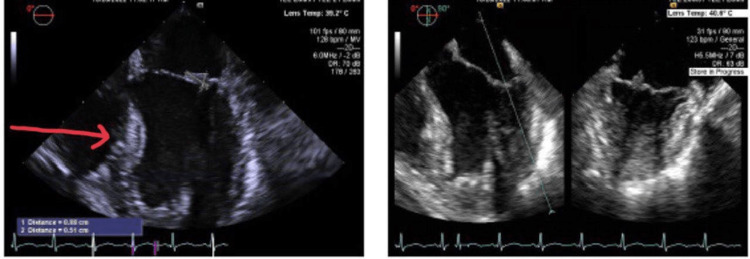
Transesophageal echocardiogram shows mobile pedunculated vegetation on the mitral valve.
